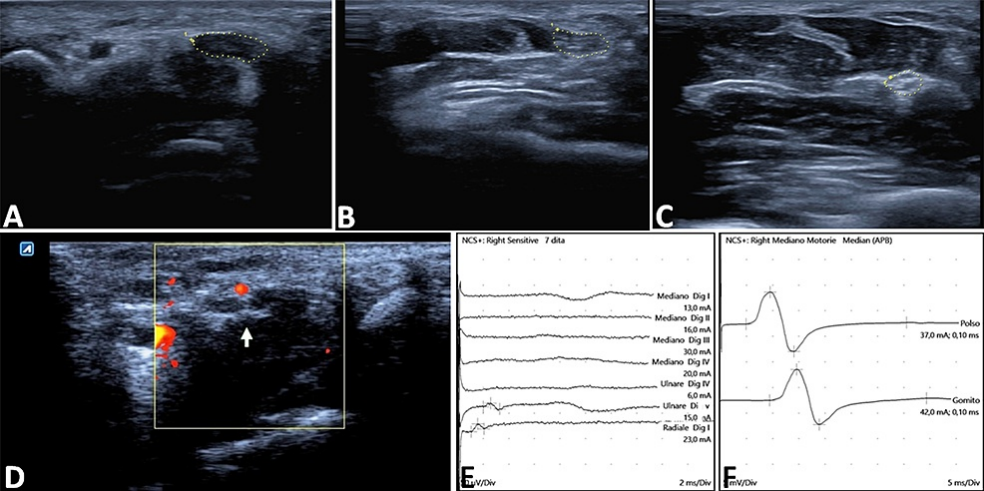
Preoperative assessment of median nerve entrapment The ultrasonographic scan of the median nerve shows a cross-sectional area (CSA) of 18 mm^2^ at the level of the pisiform bone (A), while more proximally, the median nerve (B) presents the classical “heart-shape” CSA. At the pronator quadratus level (C), the CSA and the echogenicity appear normal (CSA 8 mm^2^) with a wrist-to-forearm ratio >1.4 mm^2^. Ultrasonographic imaging could also identify the vascular structures by using the Doppler effect (D). The corresponding nerve conduction studies show a not recordable sensory conduction (E), with an increased distal latency of 4.9 ms (F).
